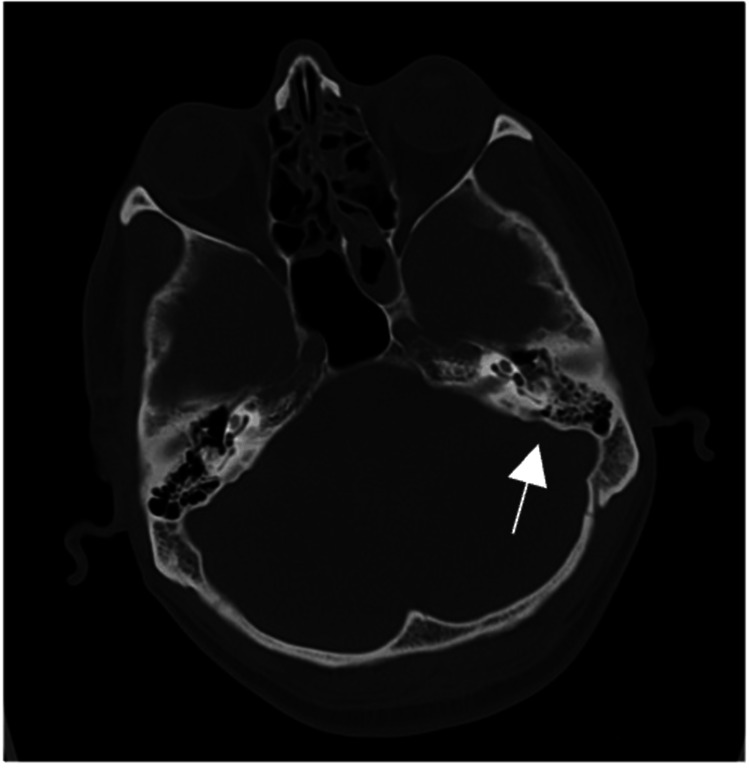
Axial view of brain CT showing opacification left mastoid air cells, possibly related to old mastoiditis or trauma
